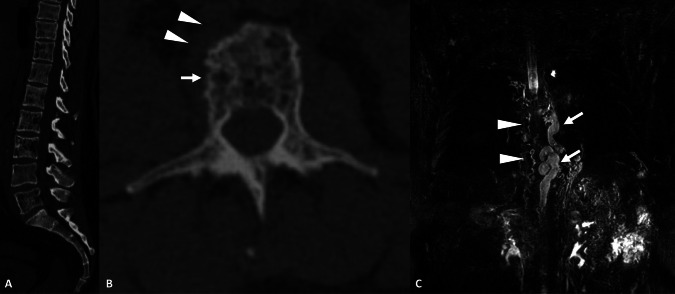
Work-up of osteolytic lesions of the spine in a 62-year-old female revealing primary diffuse lymphangiectasis. A Noncontrast CT, sagittal view. Diffuse patchy osteolytic lesions of the spine demonstrating fat attenuation density. B Noncontrast CT, axial view. Areas of cortical erosions with a moth-eaten appearance (arrow). A nonspecific prevertebral tissular mass is also identified (arrowheads). C 3D FRFSE T2w, MIP reconstruction (20 mm), coronal view revealing paravertebral lymphangiectasis (arrowheads), corresponding to the prevertebral tissular mass on CT, and a massive dilation of the thoracic duct (arrows)
